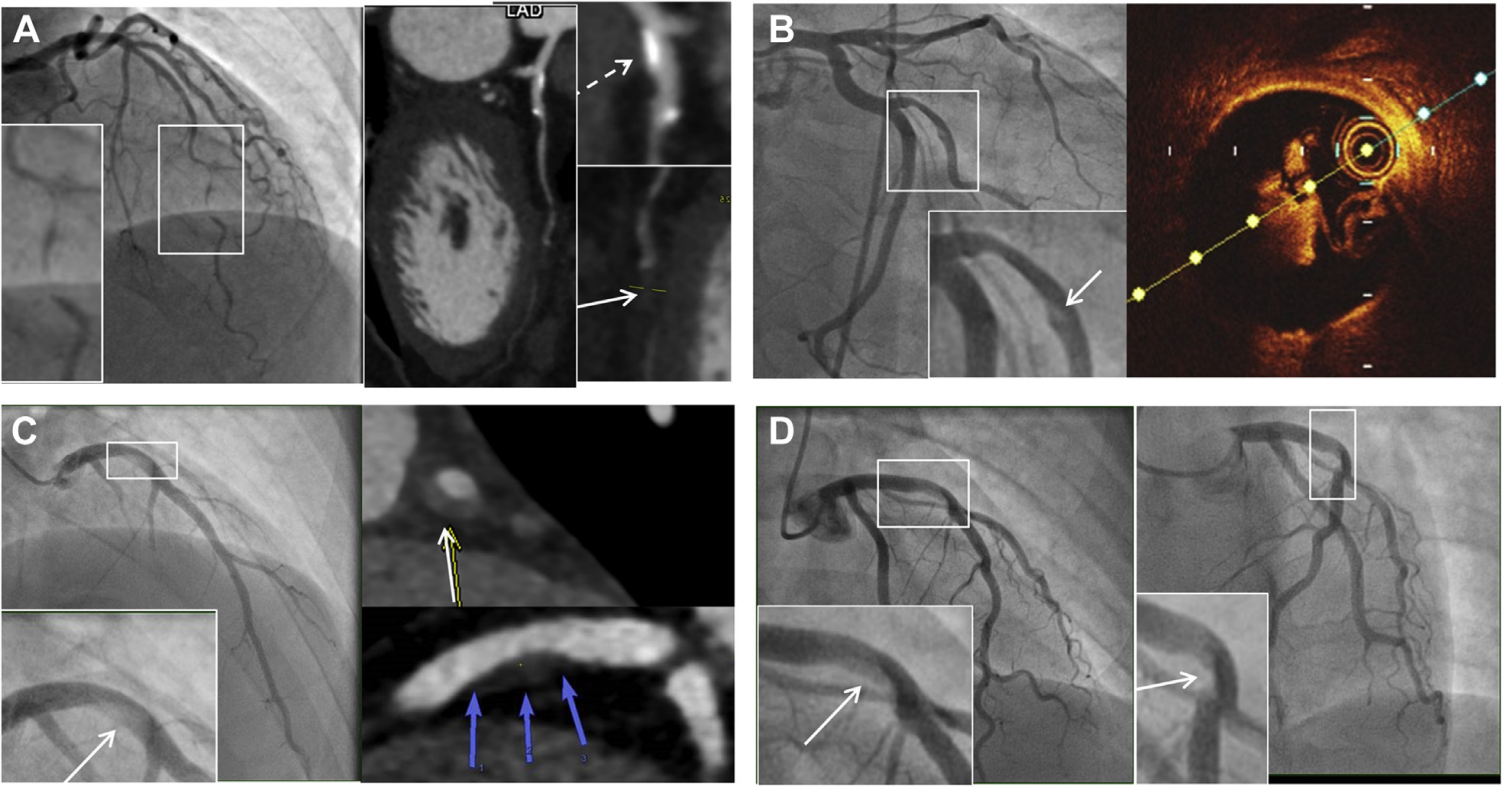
Atherosclerosis Versus Spontaneous Coronary Artery Dissection (A) A patient with angiographic appearances in keeping with Type 2a SCAD (box) but who continued to experience typical exertional angina at follow-up triggering reassessment by CTCA. This confirmed mixed calcific atherosclerotic plaque (dotted arrow) with persisting stenosis (arrow). (B) A stenosis with downstream filling defect (box and arrow) in the obtuse marginal branch of the circumflex. OCT imaging suggests this appearance is due to a tongue of organized thrombus arising from an area of plaque erosion extending within the downstream lumen. (C) A young man with no cardiovascular risk factors who developed chest pain during sport. Angiography showed a hazy linear abnormality in the mid left anterior descending coronary artery (LAD) (box). Computed tomographic angiography demonstrated positively remodeled lipid-rich atherosclerotic plaque. A similar angiographic ambiguous case (D) occurring in a young female patient a few weeks postpartum. No intracoronary imaging was performed.
